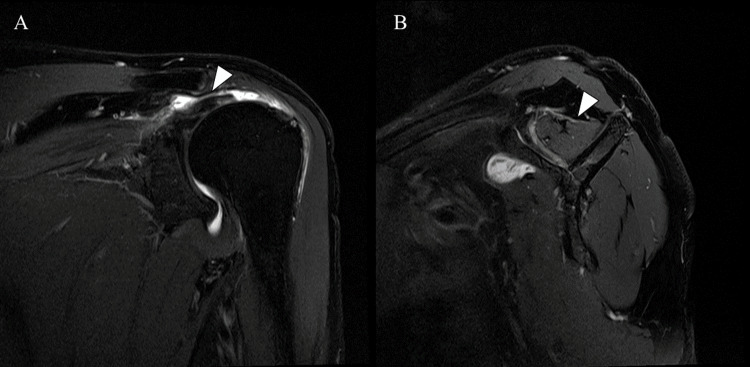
Preoperative magnetic resonance imaging of the left shoulder (A) Fat-suppressed T2-weighted images obtained in the oblique coronal plane shows moderate rotator cuff tears at the level of the supraspinatus tendon; (B) Fat-suppressed T2-weighted images obtained in the sagittal plane showing mild fatty infiltration of the supraspinatus muscle.
